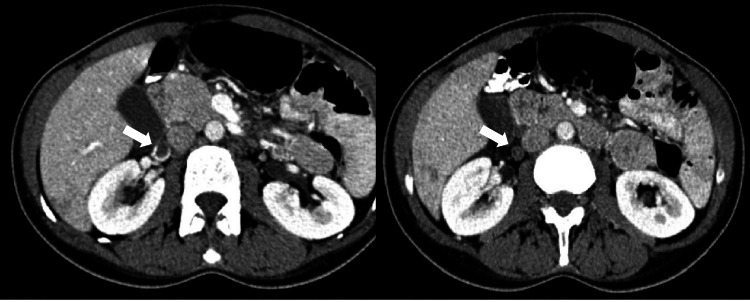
Contrast-enhanced CT (axial) Axial post-contrast CT images at two different levels in a 39-year-old female patient outside the peripartum period demonstrate a distended right ovarian vein (white arrows) with a luminal filling defect and marginal blood flow. This patient was treated by the authors at King Abdulaziz University Hospital.
